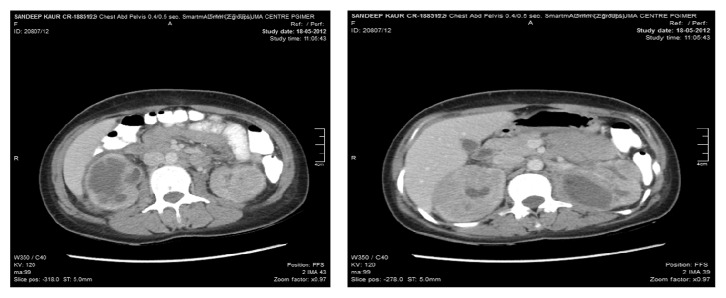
Contrast enhanced Computed Tomography scan of bilateral kidneys showing hypodense abscess like areas.
